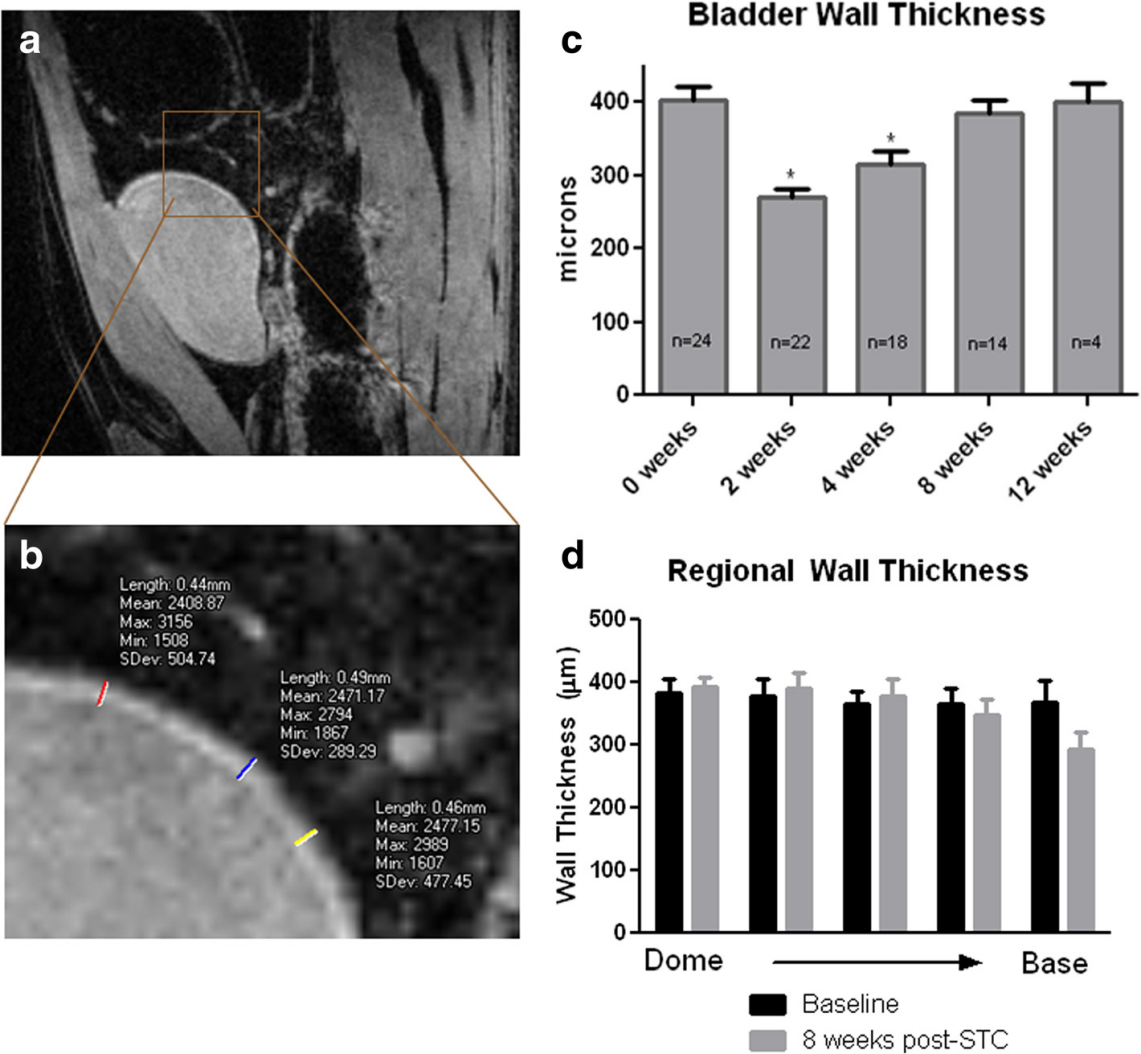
Bladder wall thickness normalizes thickness 8 weeks after STC. a Example of a sagittal view of a control (pre-STC) bladder visualized by magnetic resonance imaging (MRI), magnified in b. c 1 Way ANOVA analysis of quantified bladder wall thickness using MRI scans reveals that the bladder wall is thinner than pre-STC values 2 and 4 weeks post-STC (*P* < 0.01). The number of observations at each timepoint represent the number of successful scans at each timepoint, as instances of gating artifact did lead to some unsuccessful scans. The ratio of unsuccessful scans to total number of possible scans was 1/25, 3/25, 2/20, 1/15, and 1/5 at 0, 2, 4, 8, and 12 weeks, respectively. d Analysis of regional wall thickness shows no differences from control (*n* = 24) and 8 weeks post-STC (*n* = 14)
